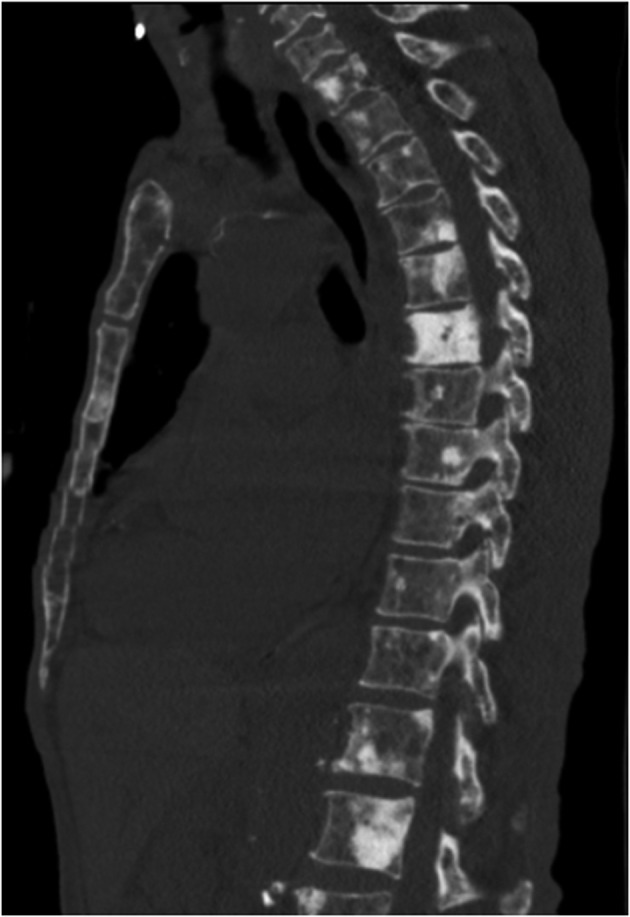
Reconstruction images of the CT chest showing multiple osteoblastic lesions in thoraco-lumbar vertebrae.
